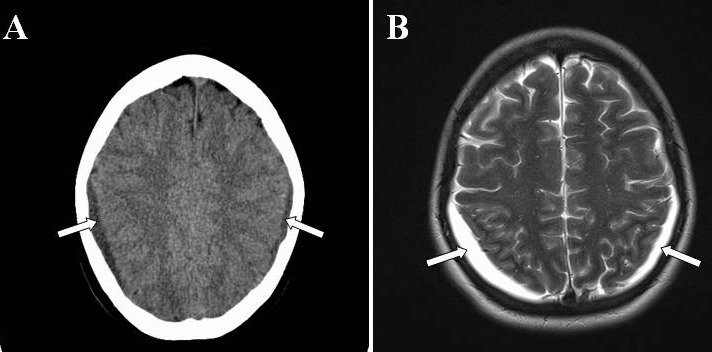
A non-contrast enhanced CT scan (A) and axial T2 weighted spin echo magnetic resonance imaging scan (B) showing bilateral subdural hematomas (marked by white arrows).
